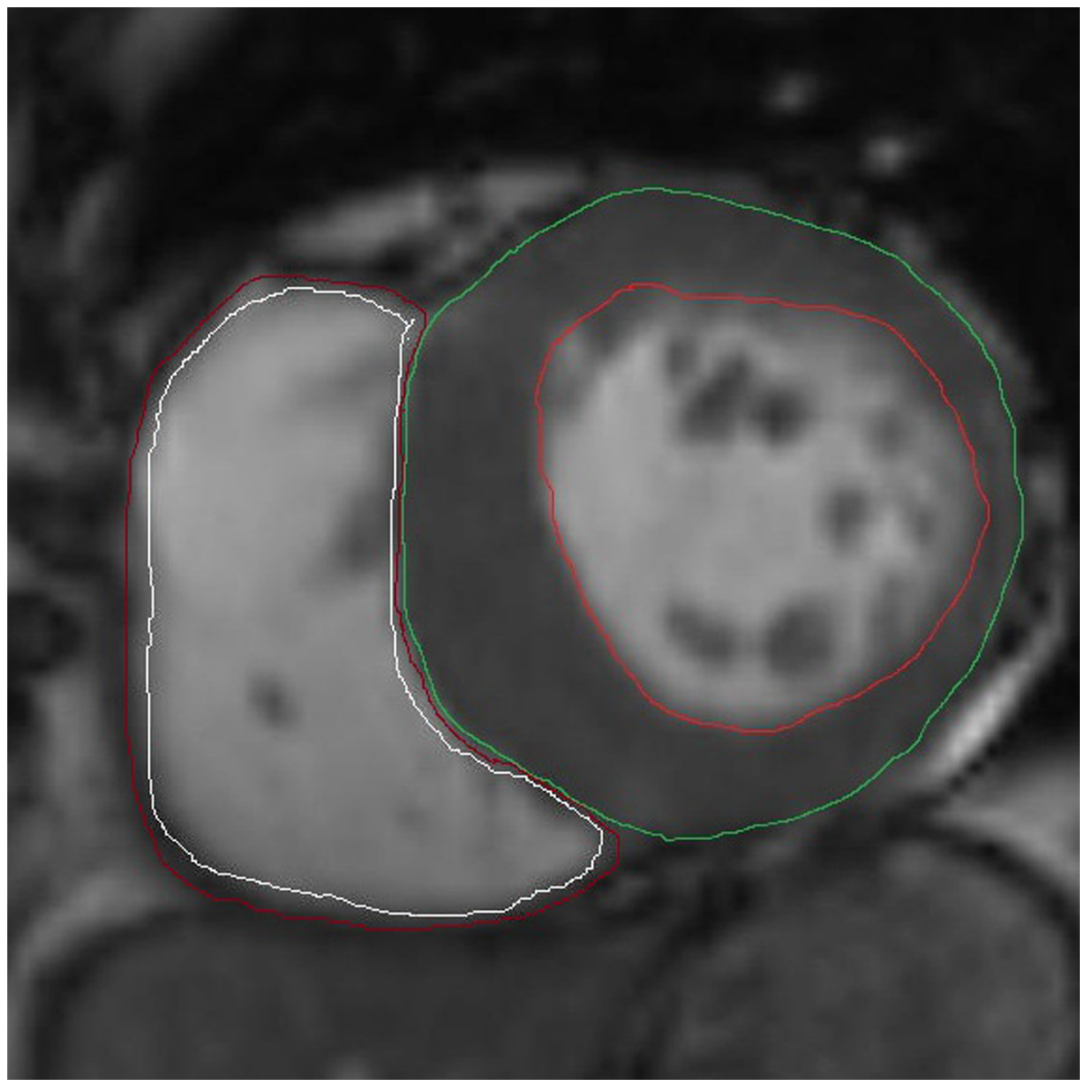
Cardiac images show an example of left and right ventricular function data obtained from the patient at end-diastole phase using MRI.
